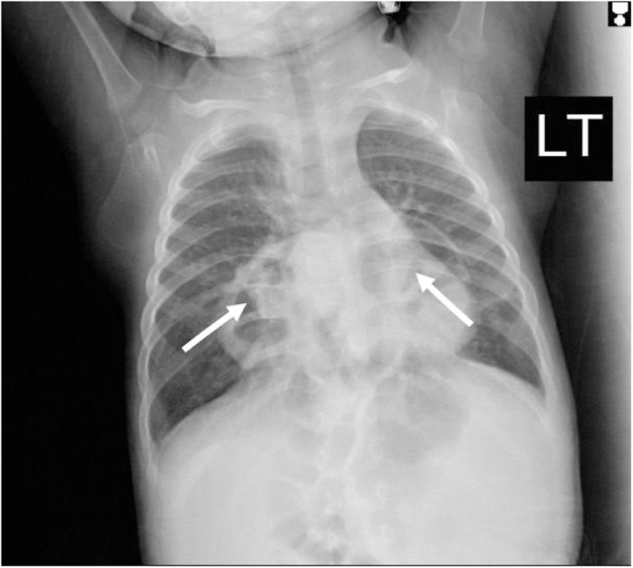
Chest X-ray (AP, supine) showing bilateral, well-defined cystic lesions are seen in the lower thorax (the white arrows), findings are suggestive of bilateral congenital diaphragmatic hernias, likely Morgagni type. No signs of consolidation or effusion.
